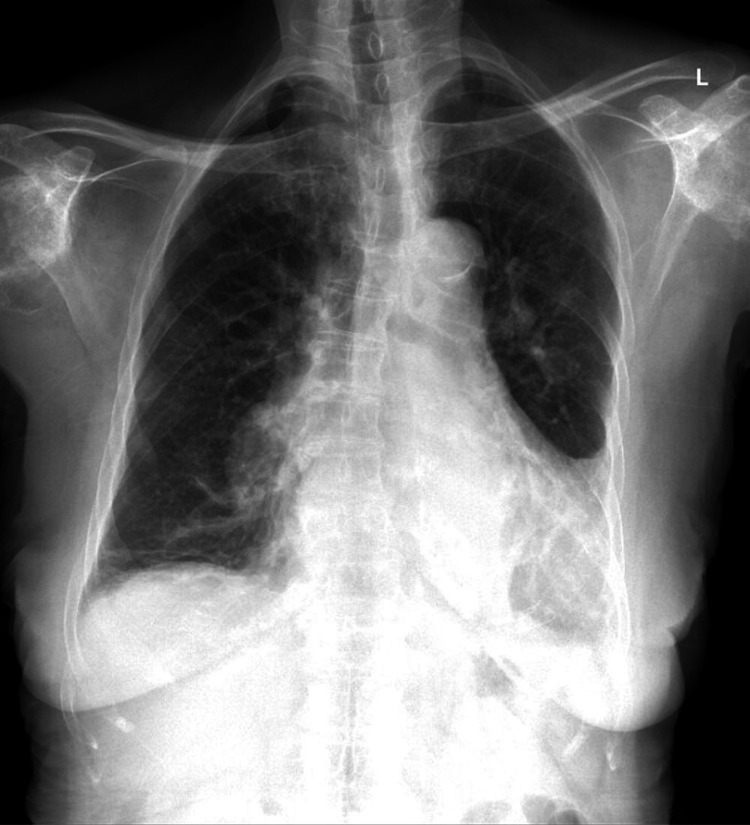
Pre-admission Chest Radiograph Pre-admission chest radiograph notable for nonspecific airspace disease within the left lower lung, retrocardiac area. Although more evident in retrospect, the right pulmonary, interlobar, and right lower lobar arteries appear dilated. This was concordant with findings on CT.
